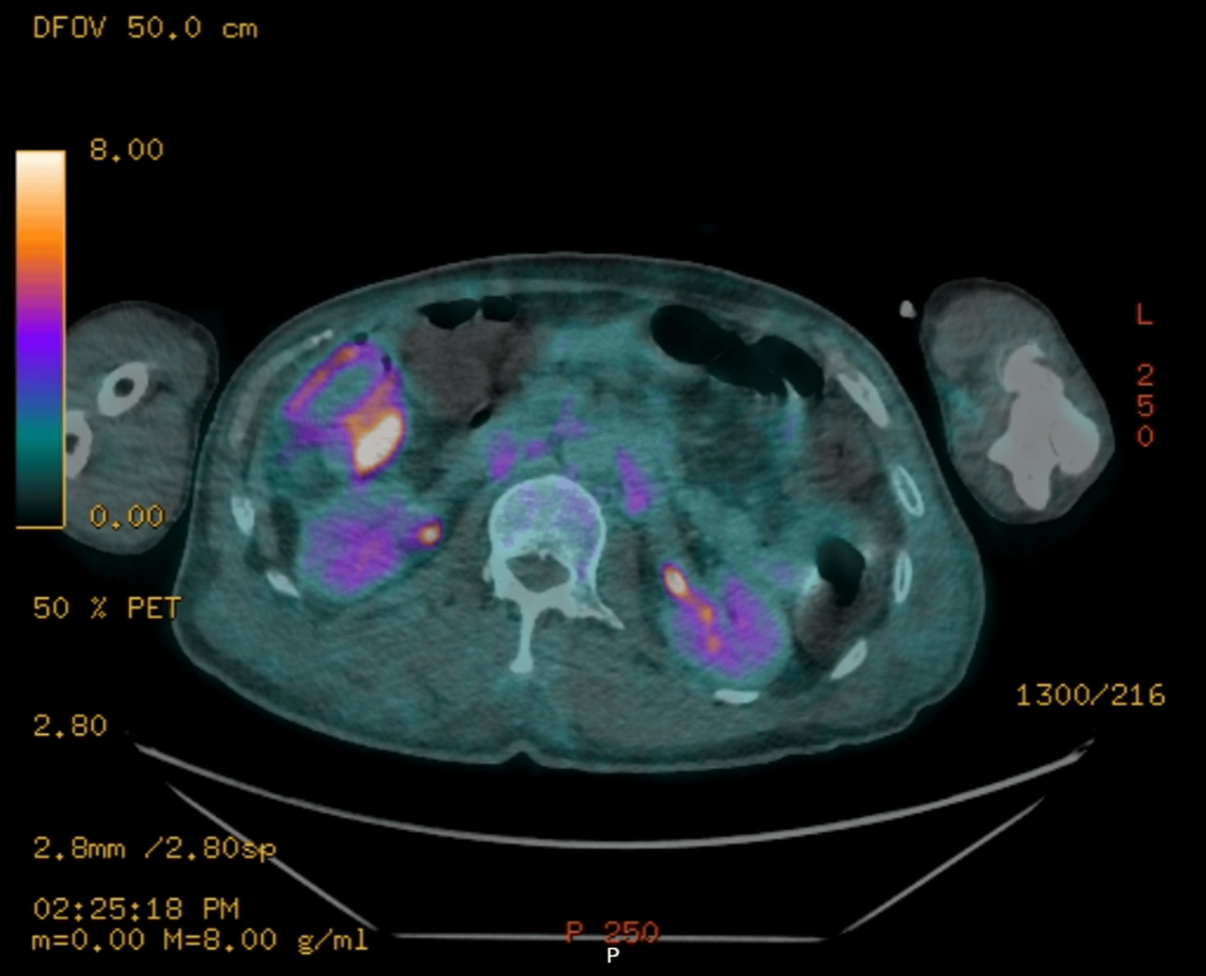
PET-CT showing a focus of FDG uptake in the distal ascending colon FDG, fluorodeoxyglucose
